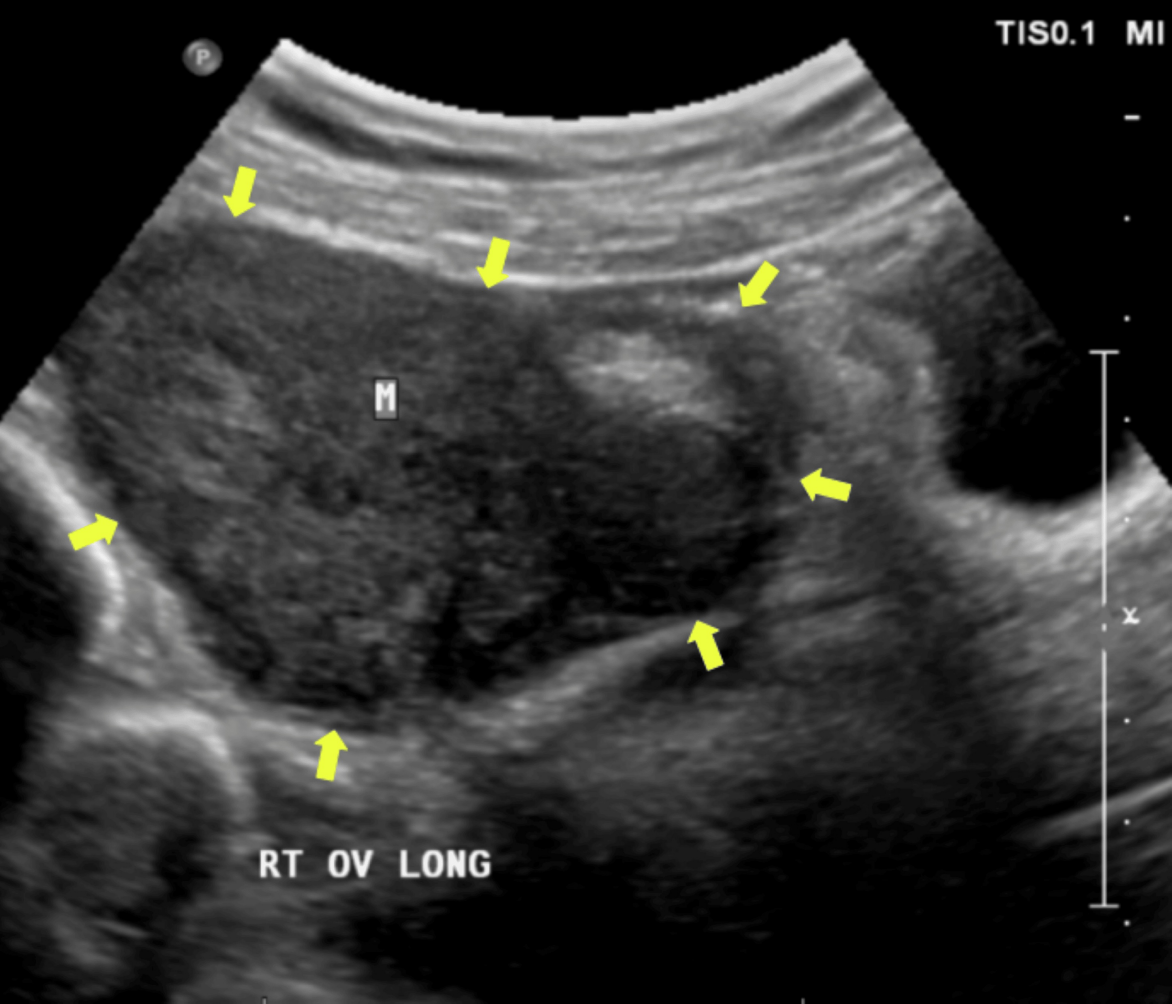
An ultrasound showing the leiomyoma in a longitudinal view. Yellow arrows showing border of leiomyoma. M - Leiomyoma
